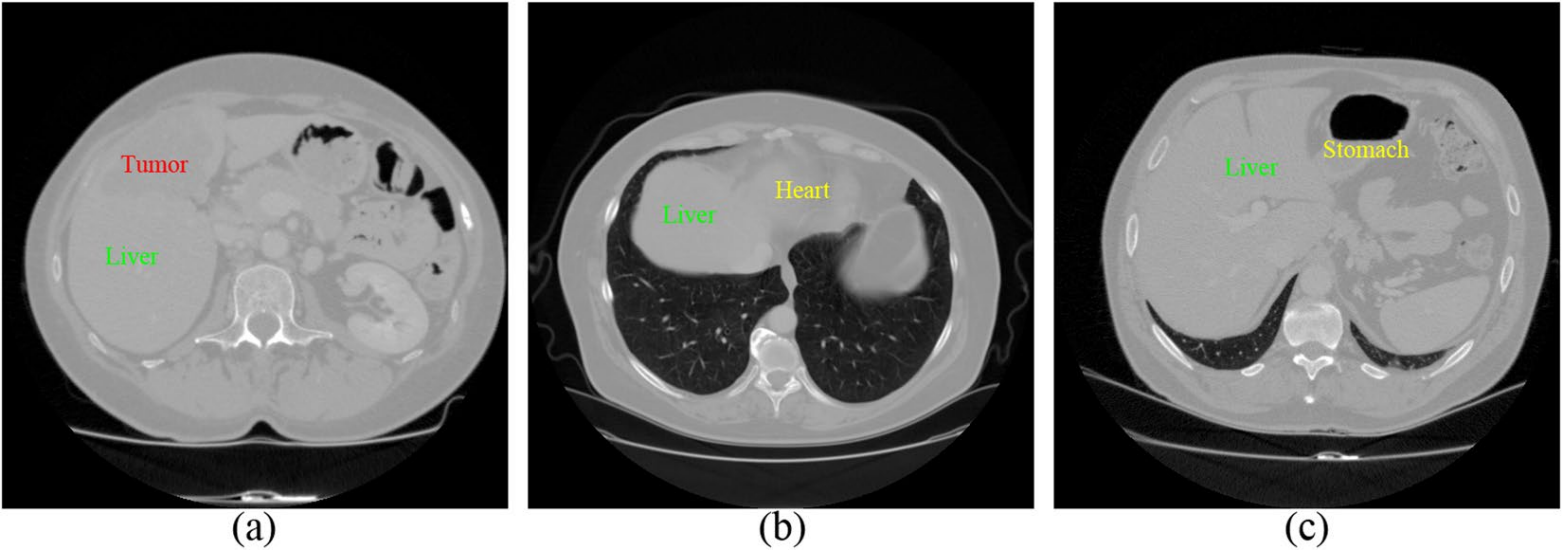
Examples of the limiting factors for liver segmentation in CT images. (a) Demonstrates intensity inhomogeneity between liver and tumor. (b) and (c) display that liver, heart, and stomach share similar intensity value.
